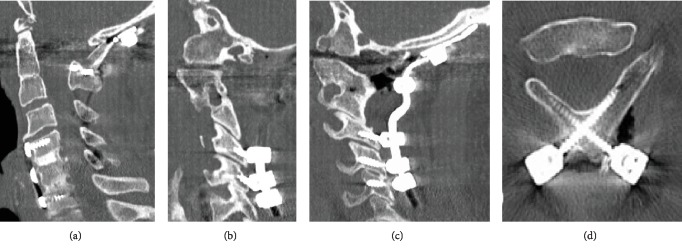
Case 1: postoperative CT. (a) The reduction of the cortical step-off between the anterior arch of C1 and the clivus with partial reduction of the odontoid. (b) Right and (c) left. C1 screws were not placed. The vertebral arteries course bilaterally below the C1 ring. Lateral mass screw placed bilaterally at C4, C5, and C6. (d) Fixation to C2-C3 vertebrae block was obtained by placing laminar screws bilaterally at C2.
